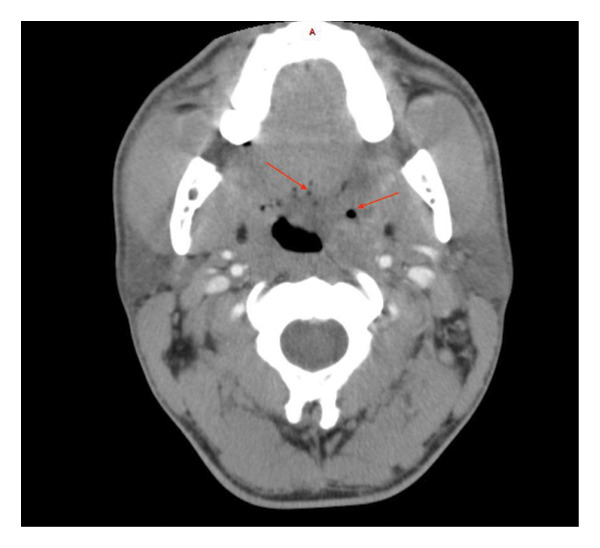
CT head with intravenous contrast demonstrating oropharyngeal lesion with areas of necrosis (red arrows).
